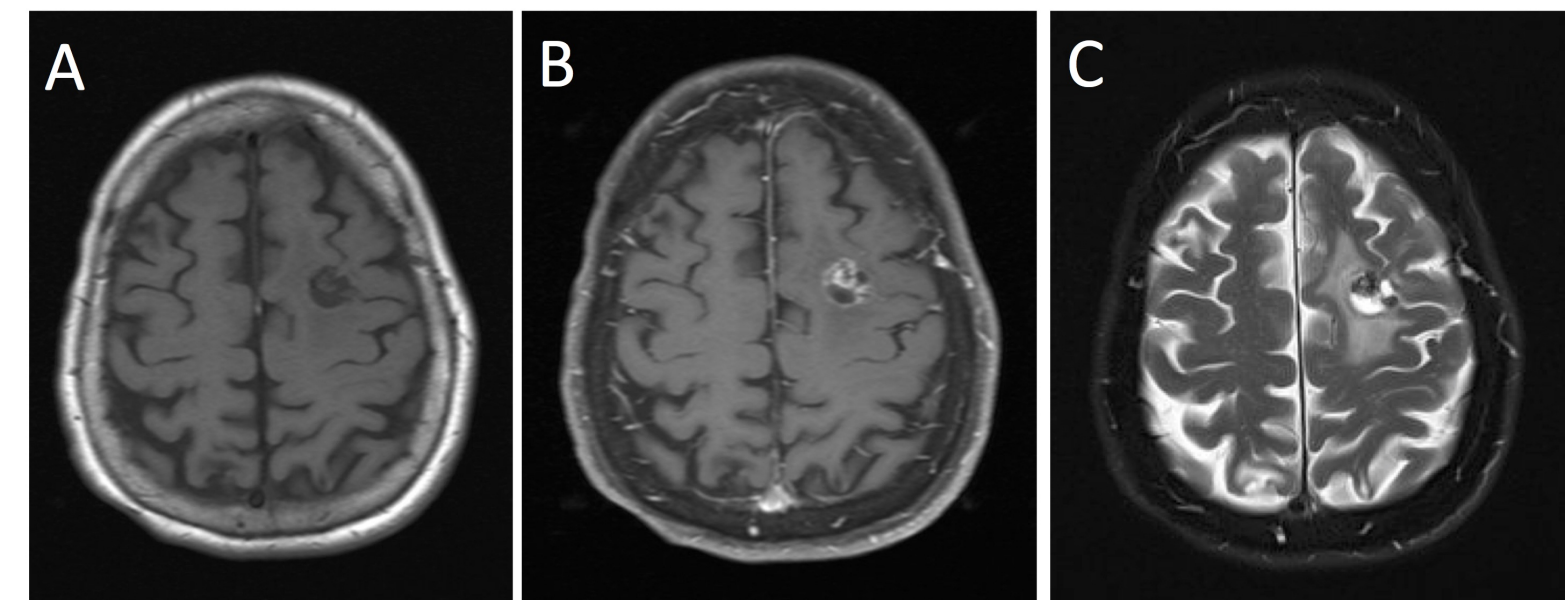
MRI evidence of lesion growth Magnetic resonance imaging (MRI). T1-weighted image pre-contrast (A) and post-contrast (B) demonstrating interval growth of the left frontal lesion. T2-weighted image showing increased area of T2 hyperintensity around the left frontal mass (C).
